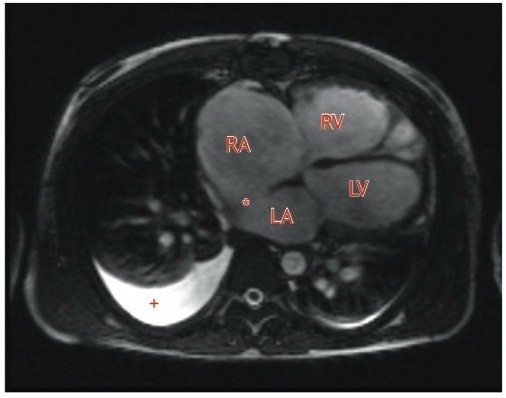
Axial T2-weighted MRI showing the grossly dilated right heart and the sinus venosus atrial septal defect (*). RA- right atrium, RV-right ventricle, LA-left atrium, LV–left ventricle. Right sided pleural effusion (+).
